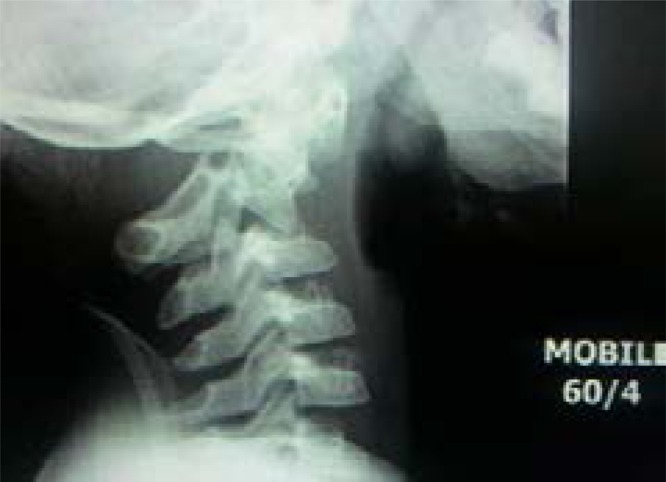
Lateral plain C-spine X-ray showing fractures of bilateral pars interarticularis with subluxation of C2 on C3.
